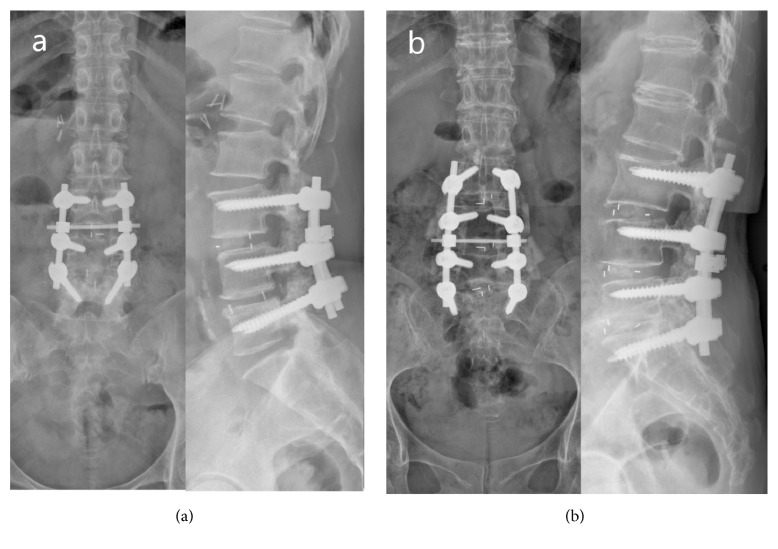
Both postoperative X-rays showed wide laminectomy decompressive procedure in degenerative thoracolumbar spine surgery (a, b). (a) 57 y/o female, degenerative spondylolisthesis with spinal stenosis of L345, who underwent wide laminectomy and instrumentation fixation and fusion. (b) 65 y/o female, degenerative disc disease of L45, L5S1 with L345S1 spinal stenosis, who underwent wide laminectomy and instrumentation fixation and fusion.
